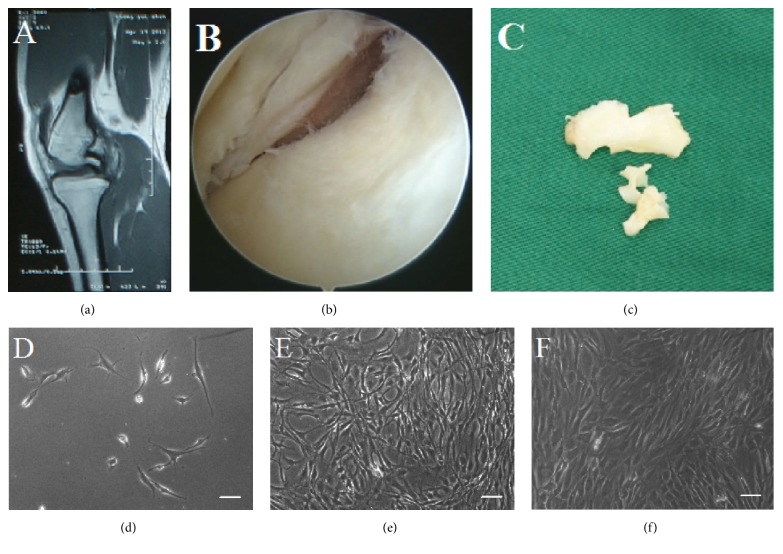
Magnetic resonance imaging (a) showing a typical bucket-handle tear of medial meniscus in sagittal view and arthroscopic examination (b) of patients with meniscal tears. Meniscal debris (c) was obtained during surgery. At 3~5 d after plating, some cells were observed to adhere to the culture dish (d). Cells took on a swirling or cluster appearance by 10–12 d (e), and they remained homogeneous until the third passage (f). Scale bar = 100 *μ*m.
